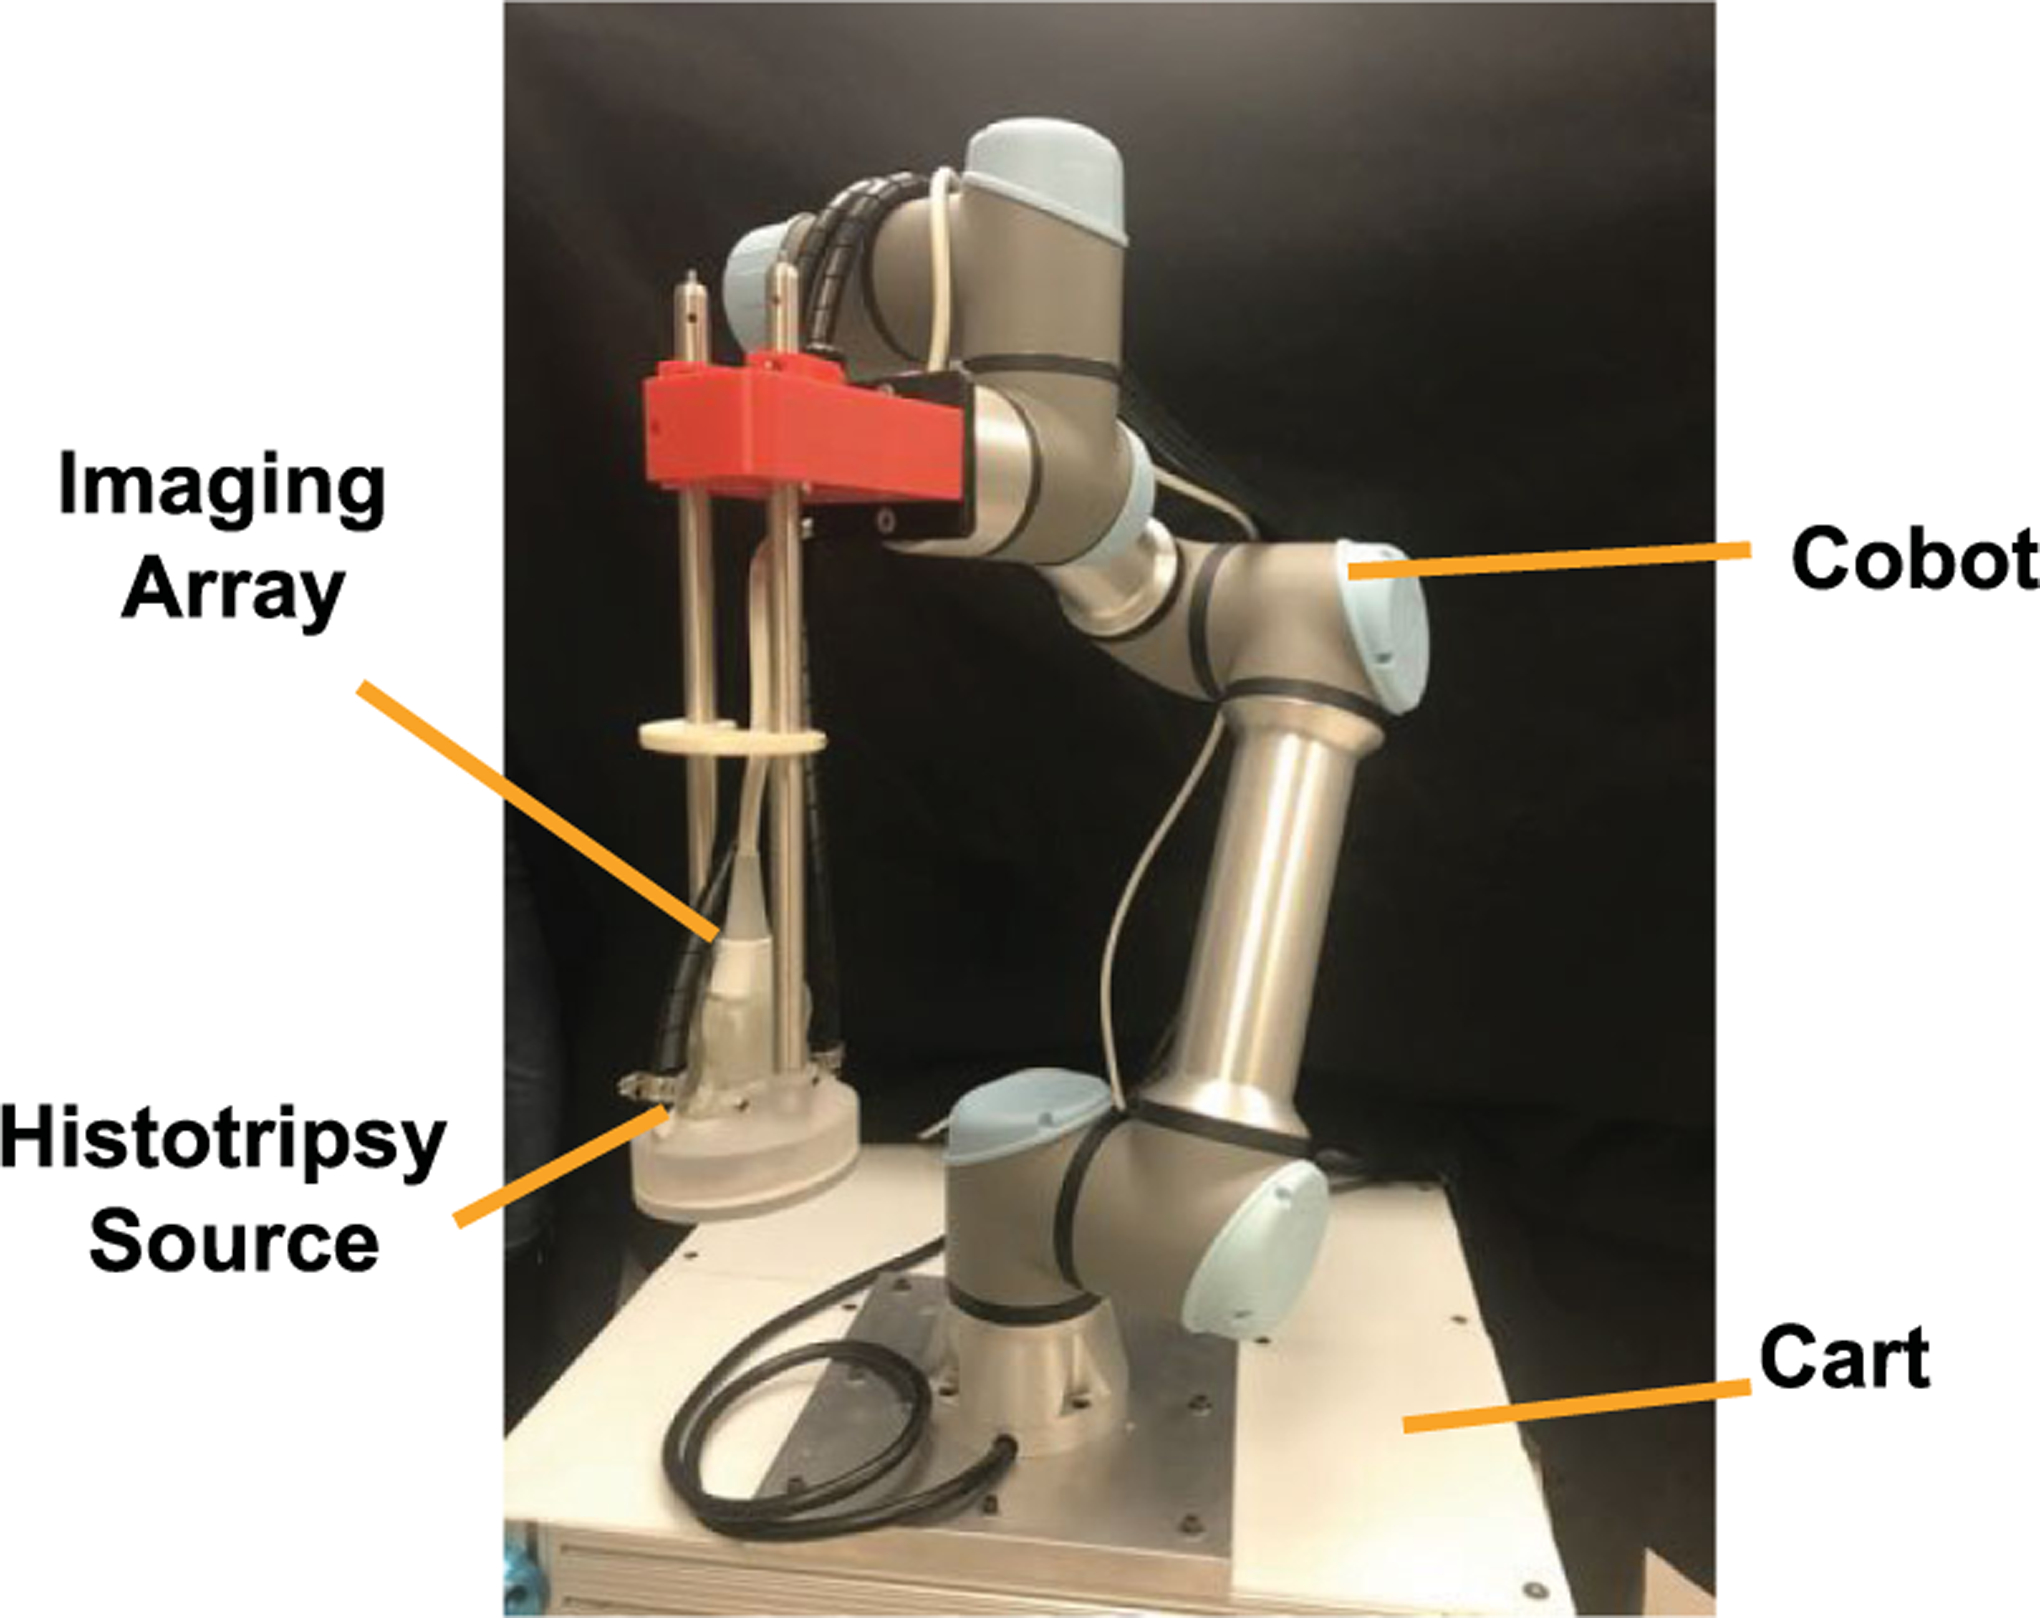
Image of histotripsy-cobot system. The histotripsy source was translated with a collaborative robot (cobot UR5e, Universal Robotics, Odesnse, Denmark). A confocal ultrasound imaging array was used to guide for the application of histotripsy pulses. The entire system was mounted on a cart to facilitate transport. Programming for the system was achieved via a Graphical User Interface (GUI).
